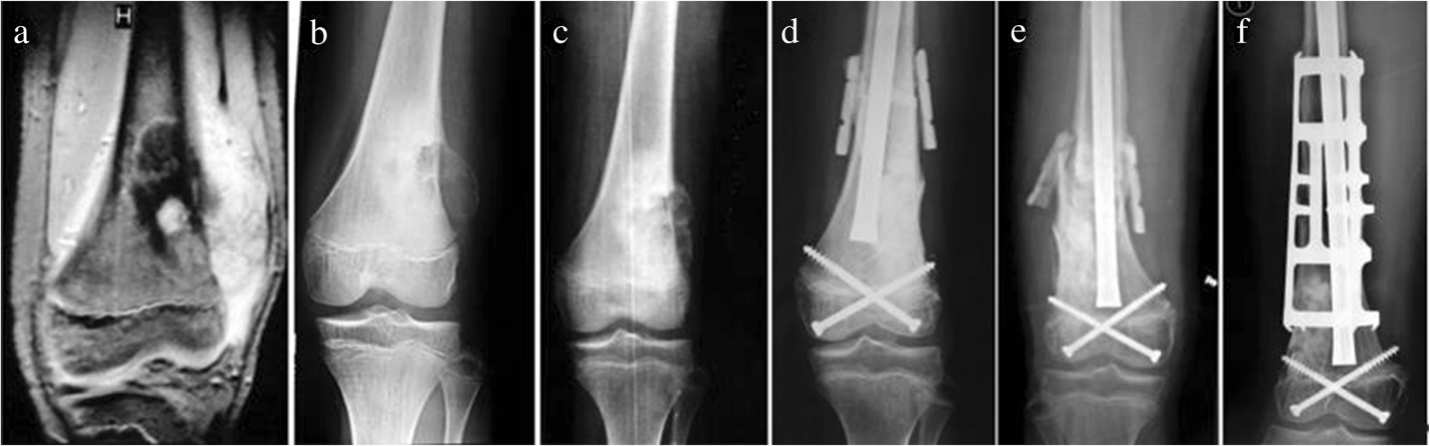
A 15-year-old male patient with osteosarcoma in left distal femur was treated with alcohol-inactivated autograft replantation with articulation preservation. a. Preoperative MRI showed intramedullary high and low mixed signal in distal femur. The lowest boarding of tumor lies 2cm over Insall line which was classified as Type I. b. Preoperative X-ray showed osteolytic bone destruction in distal femur, in accompany with severe bone destruction in lateral cortical bone, local soft tissue mass and periosteal reaction. c. Postoperative X-ray showed significantly reduced osteolytic bone destruction in distal femur, in accompany with local soft tissue mass disappearance and restoration of the continuity of the lateral periosteum. d. Two weeks after operation, X-ray showed reposition of inactivated bone with outside cortical allograft bone bridging. e. Nine months after operation, X-ray showed diaphysis fracture and bony healing in conjunction between host bone and inactivated bone. f. 30 months after reoperation, X-ray showed fully bony healing and good joint space.
